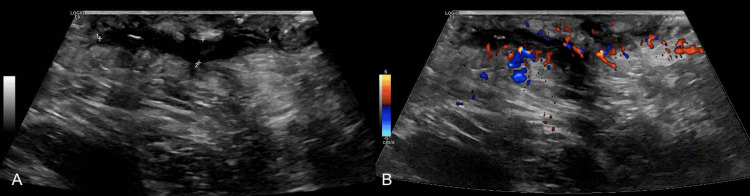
Right axillary ultrasound findings in severe hidradenitis suppurativa. (A) Gray-scale ultrasound showing an irregular subcutaneous fluid collection with internal echogenic foci and associated sinus tract formation. (B) Color Doppler image demonstrating increased peripheral vascularity consistent with active inflammation.
